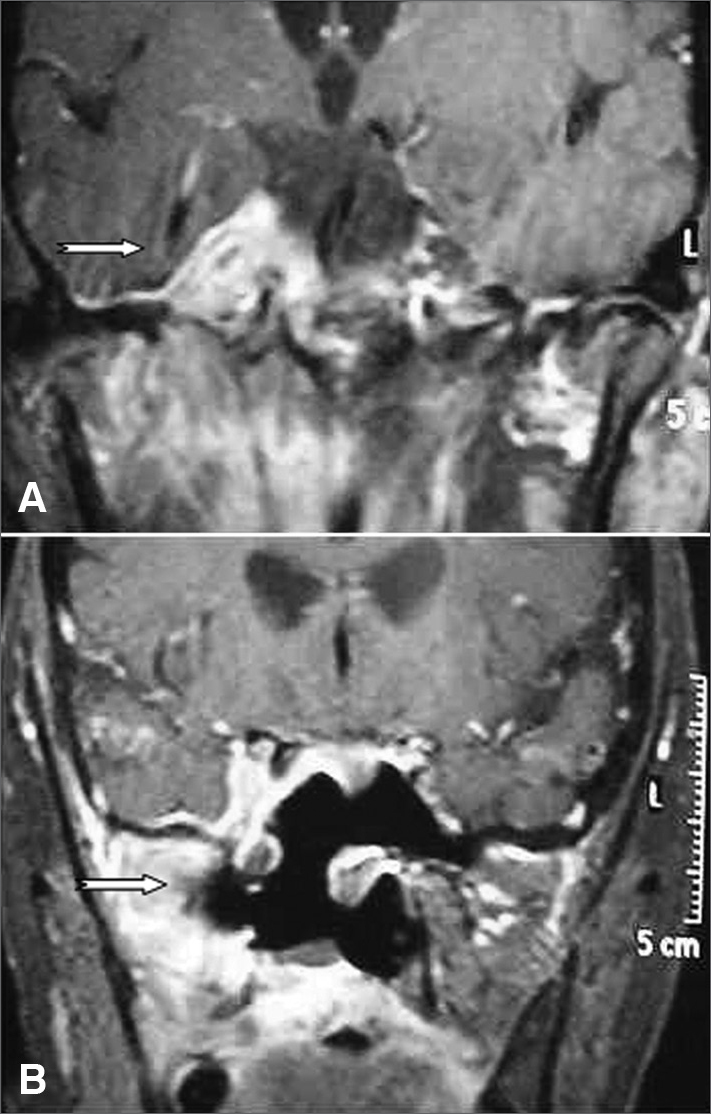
A: Preoperative skull MRI (contrast-enhanced T1). The arrow points to an expansive mass in the right cavernous sinus; B: Postoperative skull MRI (contrast-enhanced T1). The arrow points to the right cavernous sinus now without the mass.
